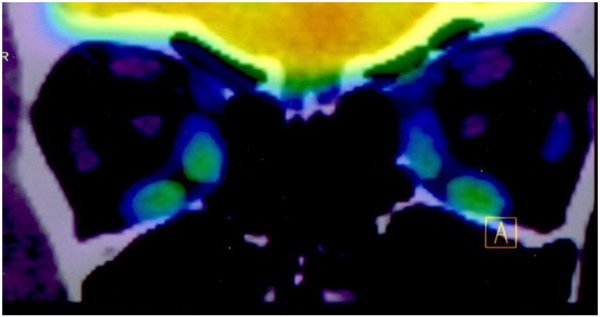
Increased FDG uptake by the inferior and medial rectus muscles on PET/CT.
